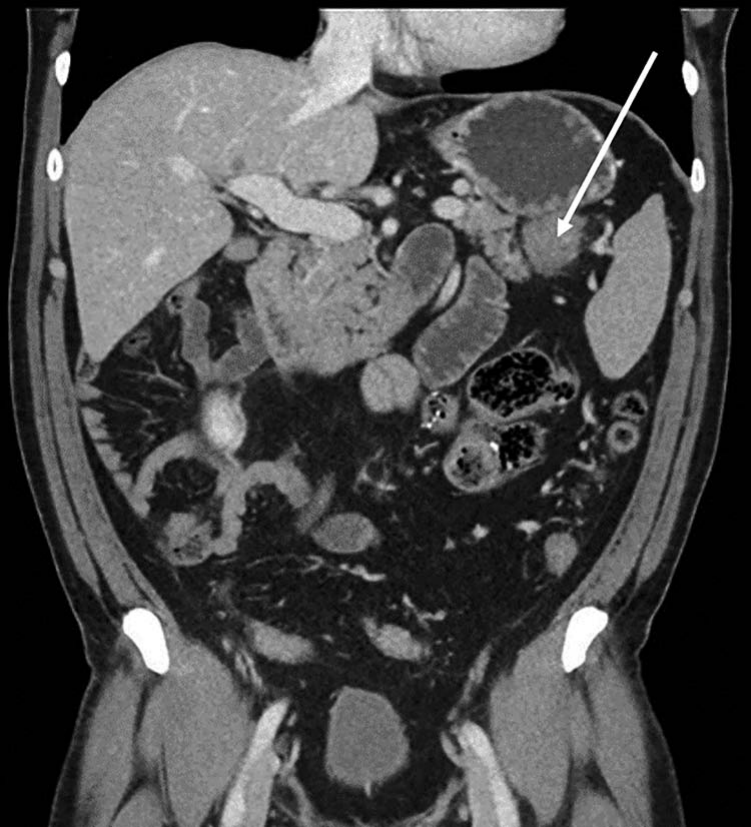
Coronal CT scan of abdomen, selected image from a pancreatic protocol, portal venous phase. A 3.7-cm mass, between the tail of the pancreas and the splenic hilum, corresponding to a peritoneal implant (arrow).
